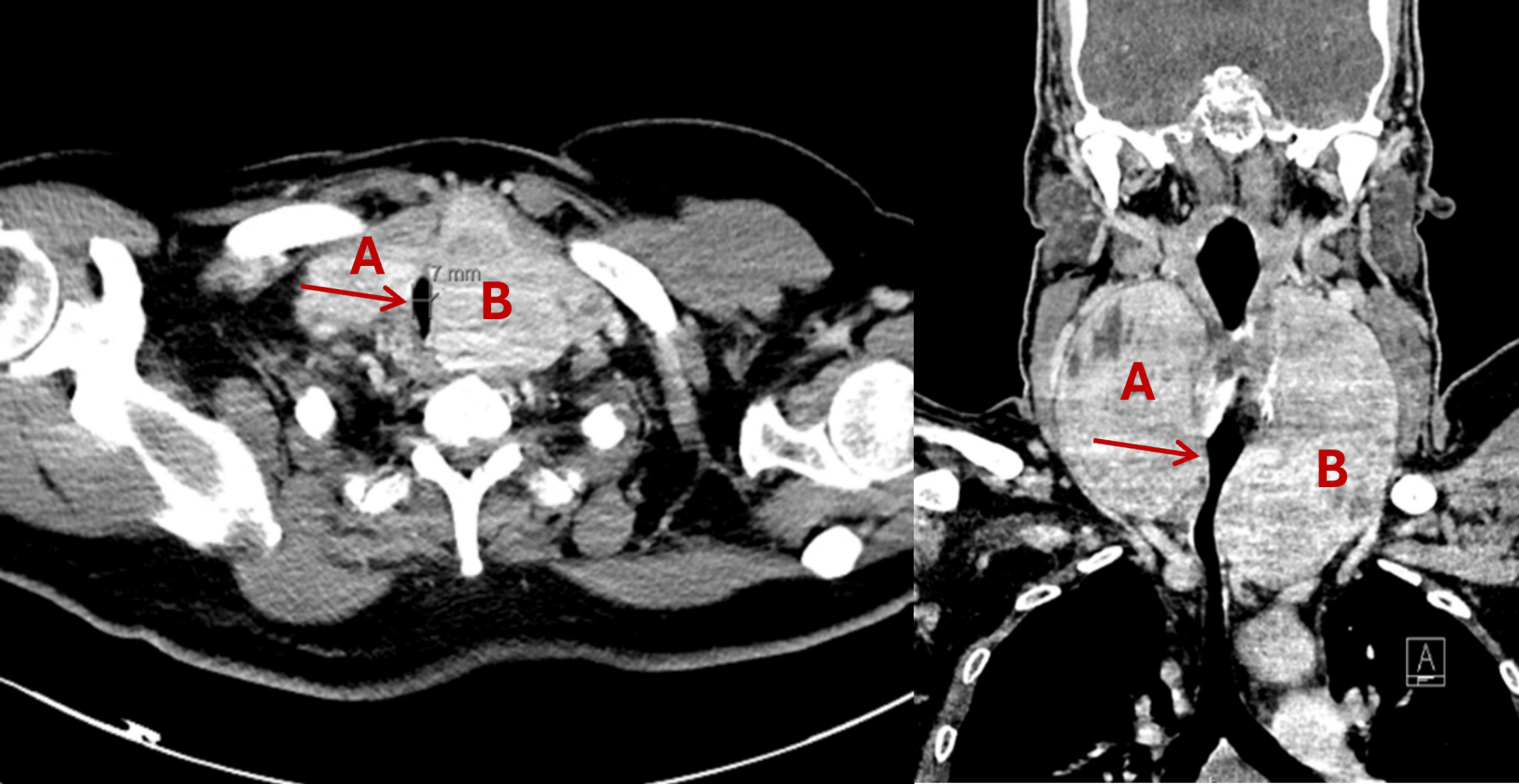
A CT scan of the patient’s neck in axial (left) and coronal (right) views A) Right thyroid lobe. (B) Left thyroid lobe. Arrows indicate tracheal lumen narrowing.
